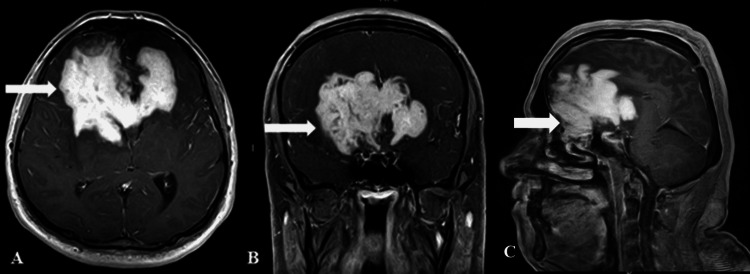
Post-contrast magnetic resonance images of the brain. Axial (A) and coronal (B) post-gadolinium T1-weighted sequences showing an avidly enhancing lesion infiltrating the corpus callosum and bilateral frontal lobes (arrows). On the sagittal image (C), the lesion can be seen infiltrating the floor of the anterior cranial fossa with extension into the ethmoid sinuses (arrow).
